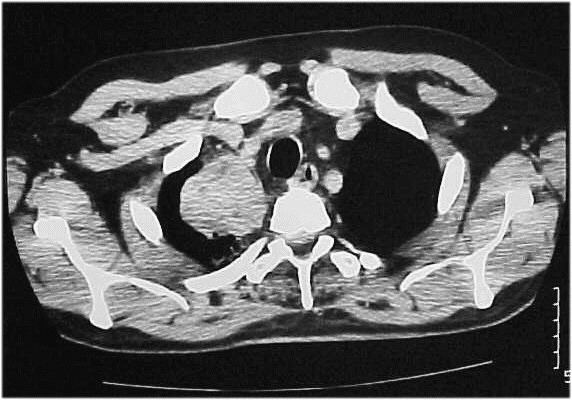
CT-scan of the chest showing a 60 × 53 mm invasive process in the right upper lobe of the lung.
